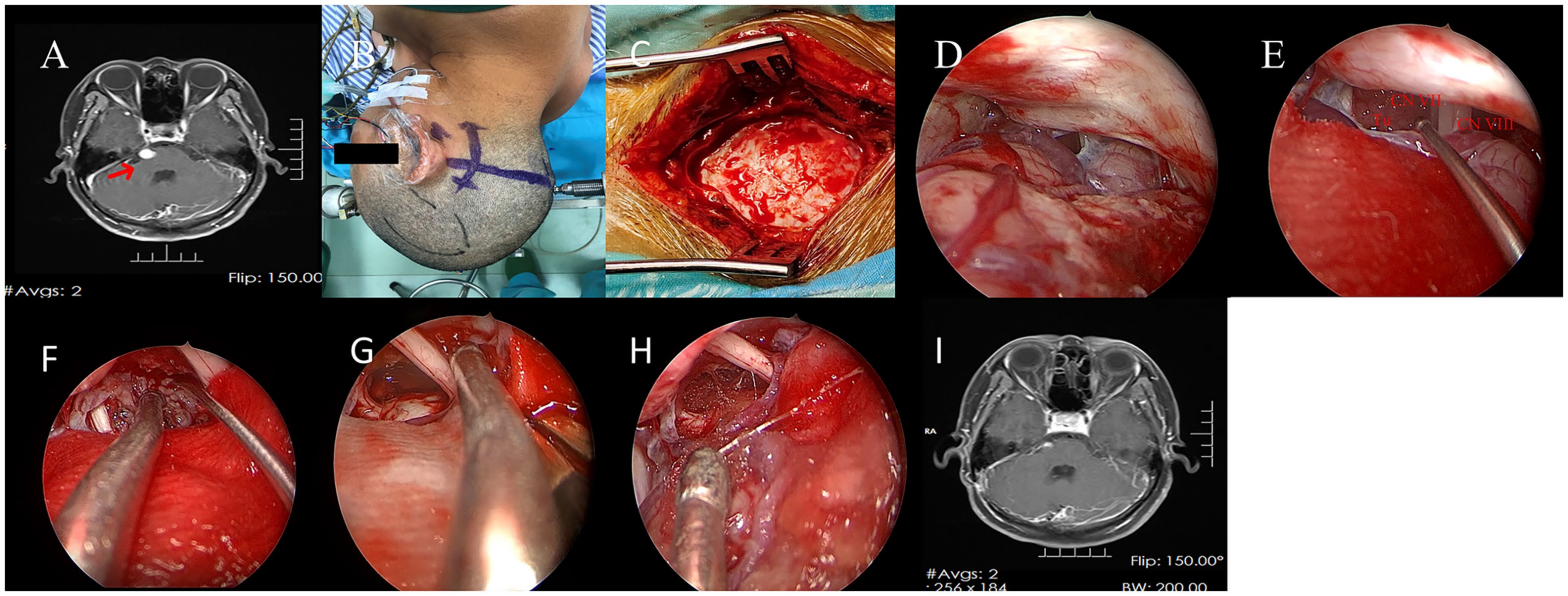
(A) Preoperative MRI revealed a mass in the patient’s right petrous apex. (B) The right retrosigmoid approach was selected. (C) The extent of the bone window. (D) Cerebrospinal fluid was slowly drained, achieving satisfactory brain relaxation. (E) Intraoperative exploration visualized the tumor, facial nerve, and vestibulocochlear nerve. (F) The tumor was gradually removed. (G) The surgical cavity after tumor resection. (H) Hemostasis was achieved using Surgicel and fluid gelatin, with no significant bleeding observed. (I) Postoperative MRI confirmed complete tumor resection.
